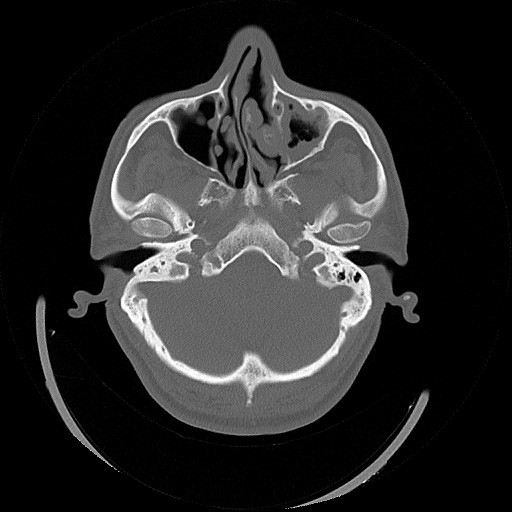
Axial paranasal computed tomography slice showing anatomical variation of superior turbinate.
